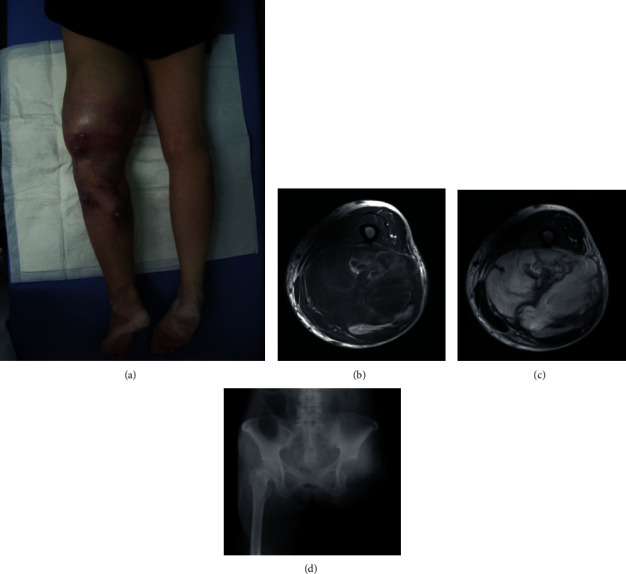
Tumor at the moment of revisiting and hip disarticulation for tumor excision. Tumor in (a) macroscopic image, (b) T1-weighted, and (c) T2-weighted MRI. (d) Plain radiograph after left hip disarticulation.
